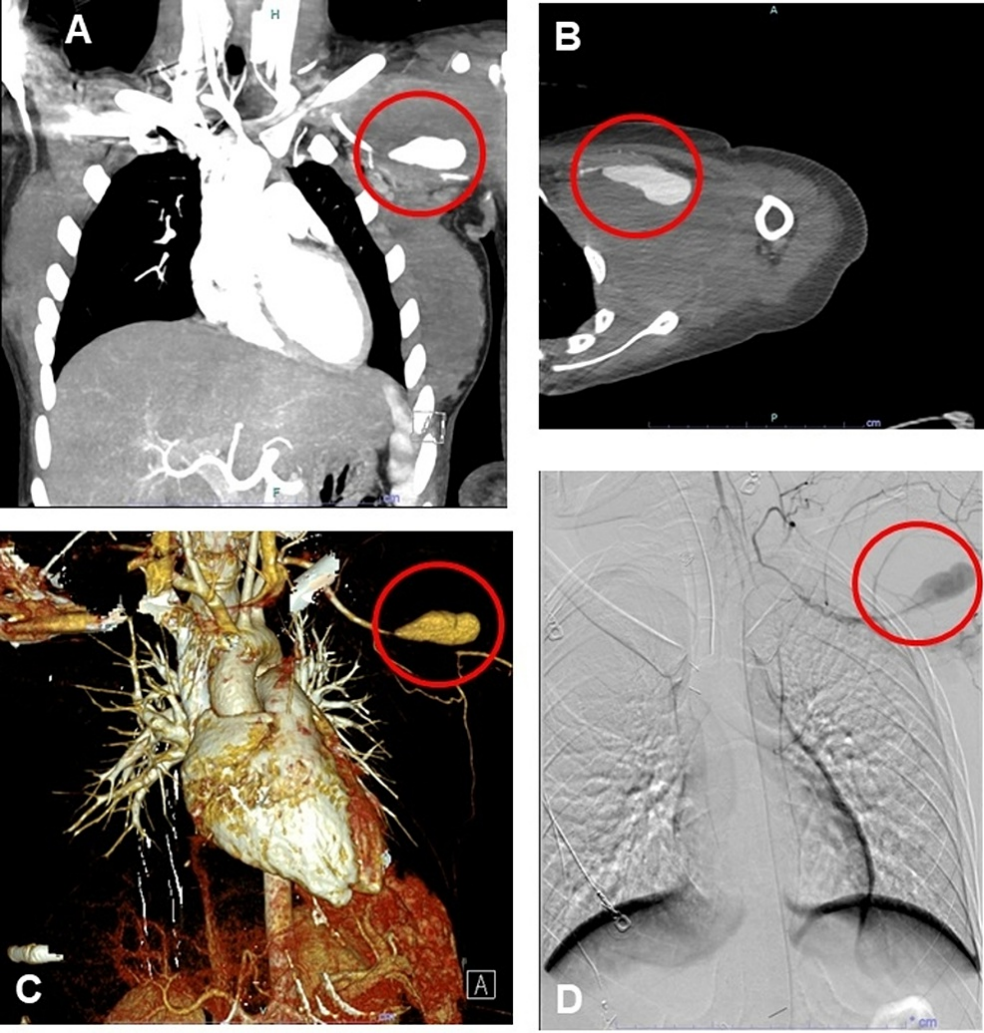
Imaging of the left subclavian artery pseudoaneurysm (circled) measuring 5cm x 1.8cm compressing the brachial plexus through various modalities three months after the initial traumatic event. (A) CT angiography of the thorax. (B) CT angiography of the left upper extremity. (C) Three-dimensional reconstruction of the angiography. (D) Intraoperative fluoroscopy of the left subclavian artery.
